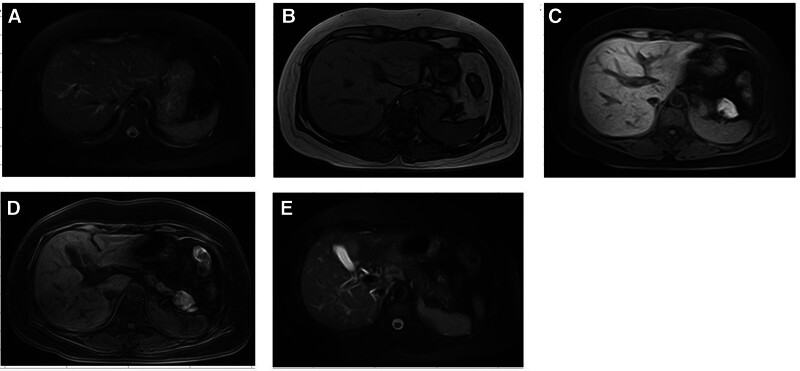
Abdominal MRI findings upon admission. MRI = magneticresonance imaging.
